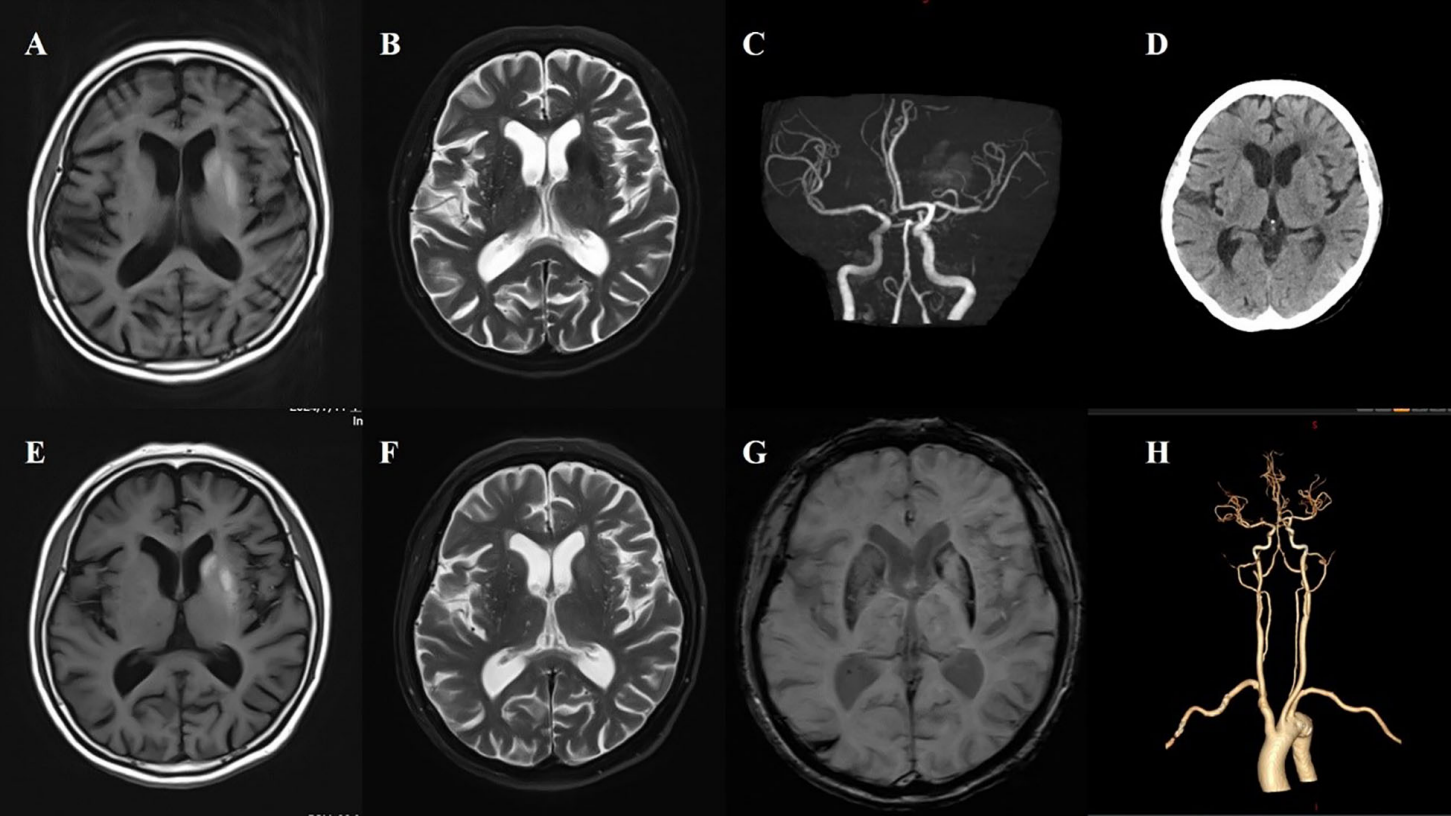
Case 1. (A) The first MR T1 image demonstrated hyperintensity in the left putamen and the head of the caudate. (B) The first MR T2 image revealed hypointensity in the left putamen and the head of the caudate. (C) Brain MRA indicated mild-moderate stenosis of left MCA. (D) Brain CT showed no significant abnormalities in basal ganglia. (E) A follow-up brain MR T1 image after one month showed high signals were more pronounced in the left putamen and the head of the caudate. (F) The second brain MR T2 image revealed that the lesion on the left side changed from a low signal to a high signal and isointense. (G) SWI sequence shows asymmetric low signals in the bilateral basal ganglia (putamen and the head of the caudate), with the left side being more pronounced. (H) CTA indicated mild-moderate stenosis of left MCA and multiple intracranial vascular stenosis.
